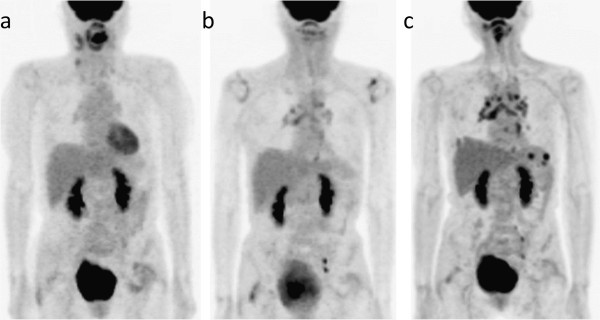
Case 3. MIP images of FDG-PET(/CT) scans that were obtained at the time of the diagnosis of the malignancy (a) and 9 months (b) and 2 years (c) after the completion of antineoplastic therapy.
